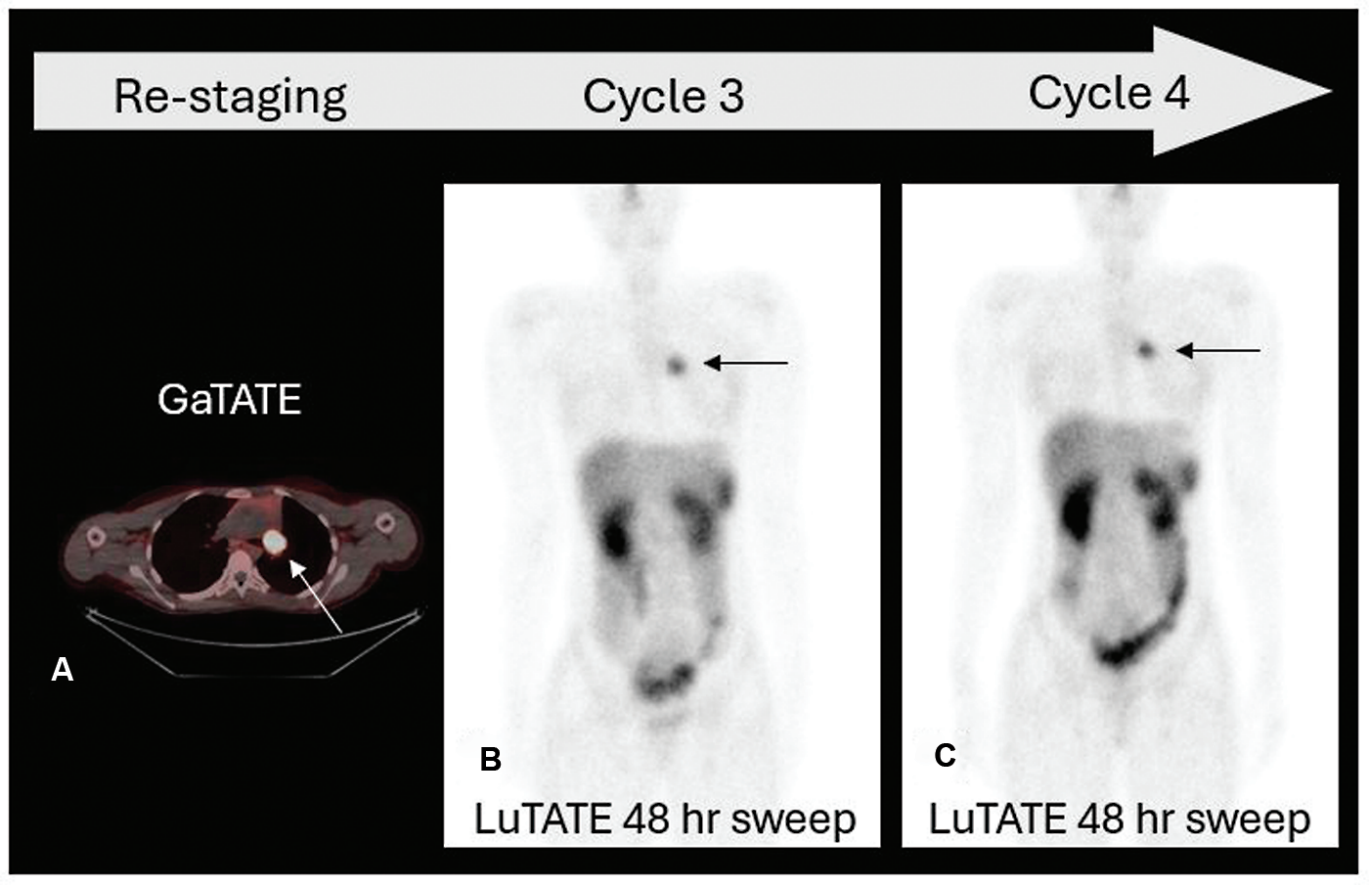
Axial GaTATE PET CT prior to further radionuclide therapy (
